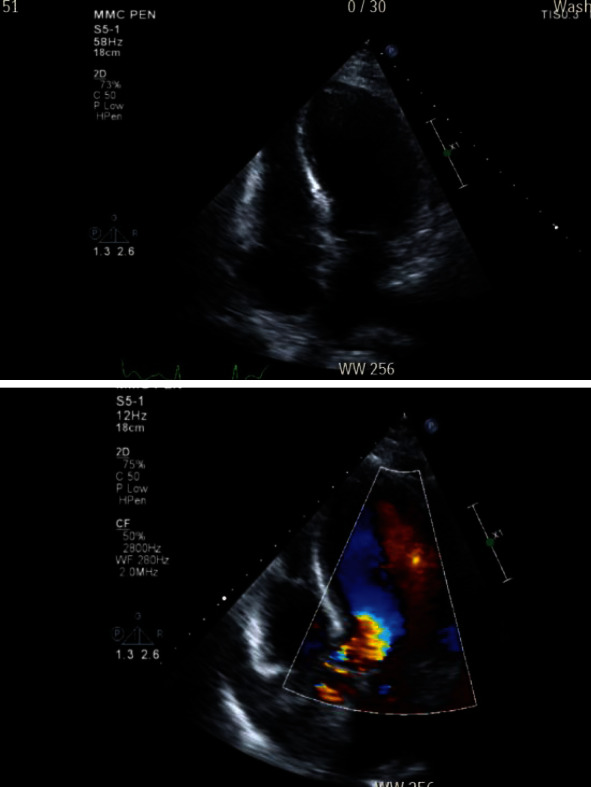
Echocardiography showing left ventricular ballooning.
